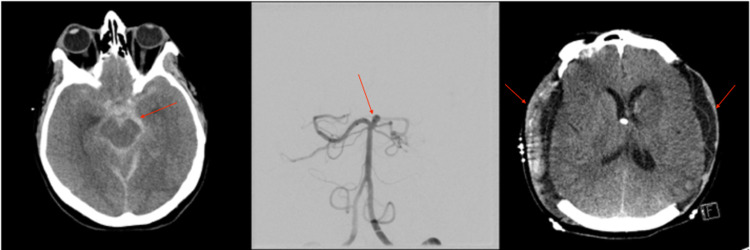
Imaging related to the clinical course of patient 2. (A) Head CT obtained on presentation demonstrated SAH secondary to a ruptured basilar tip aneurysm. (B) Angiogram obtained after presentation demonstrating a basilar tip aneurysm. (C) Post-operative head CT after bilateral DCHC for refractory elevated ICP. SAH, subarachnoid hemorrhage; DCHC, decompressive hemicraniectomy; ICP, intracranial pressure
